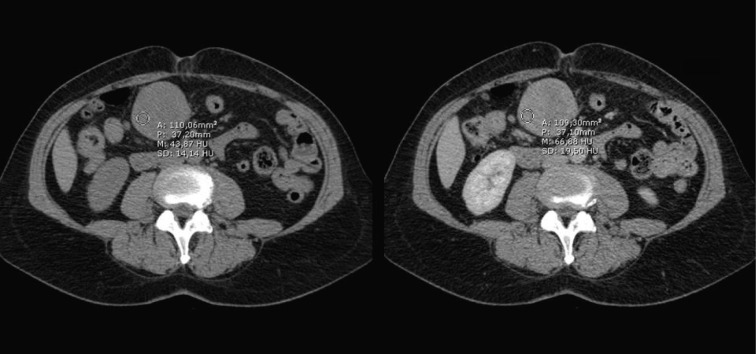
CT-scan showing on the left a non contrast image of the lesion and on the right a post contrast image showing slight enhancement, depicted as the drawn circle with the mean Hounsfield units.
